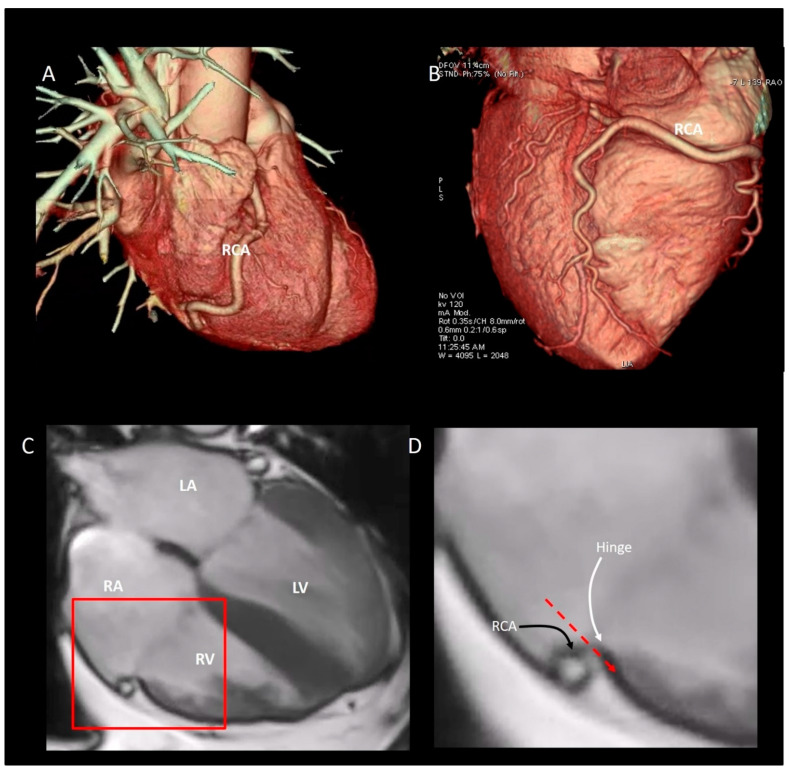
CT scan volume rendering showing the undulating course of right coronary artery (RCA). The RCA is visualized from lateral (A) and posterior (B) perspectives. (C) CMR four chamber view. (D) Magnified image of the structures inside the red square of (C), showing the close proximity between the hinge line of leaflet (white curved arrow) and the RCA (black curved arrow). The red dotted line marks the direction of the anchors used for the percutaneous implant of a prosthetic ring. RA = right atrium; LA = left atrium; RV = right ventricle; LV = left ventricle.
